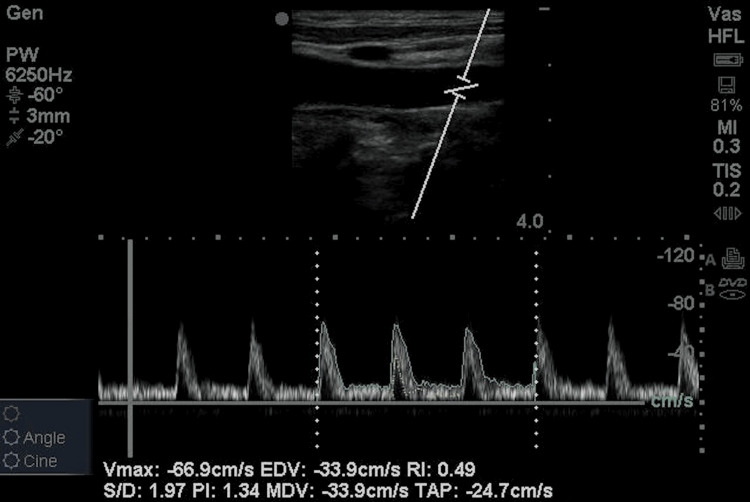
Common carotid artery spectral Doppler The  green  tracing  represents the TAMAX, also known as TAP; while the yellow tracing represents the TAMEAN; TAMAX being more than TAMEAN TAMAX: time-averaged maximum velocity; TAP: time-averaged peak velocity; TAMEAN: time-averaged mean velocity; RI: resistive index; PI: pulsatility index; EDV: end diastolic velocity; MDV: mean diastolic velocity; PW: pulsed-wave Doppler
